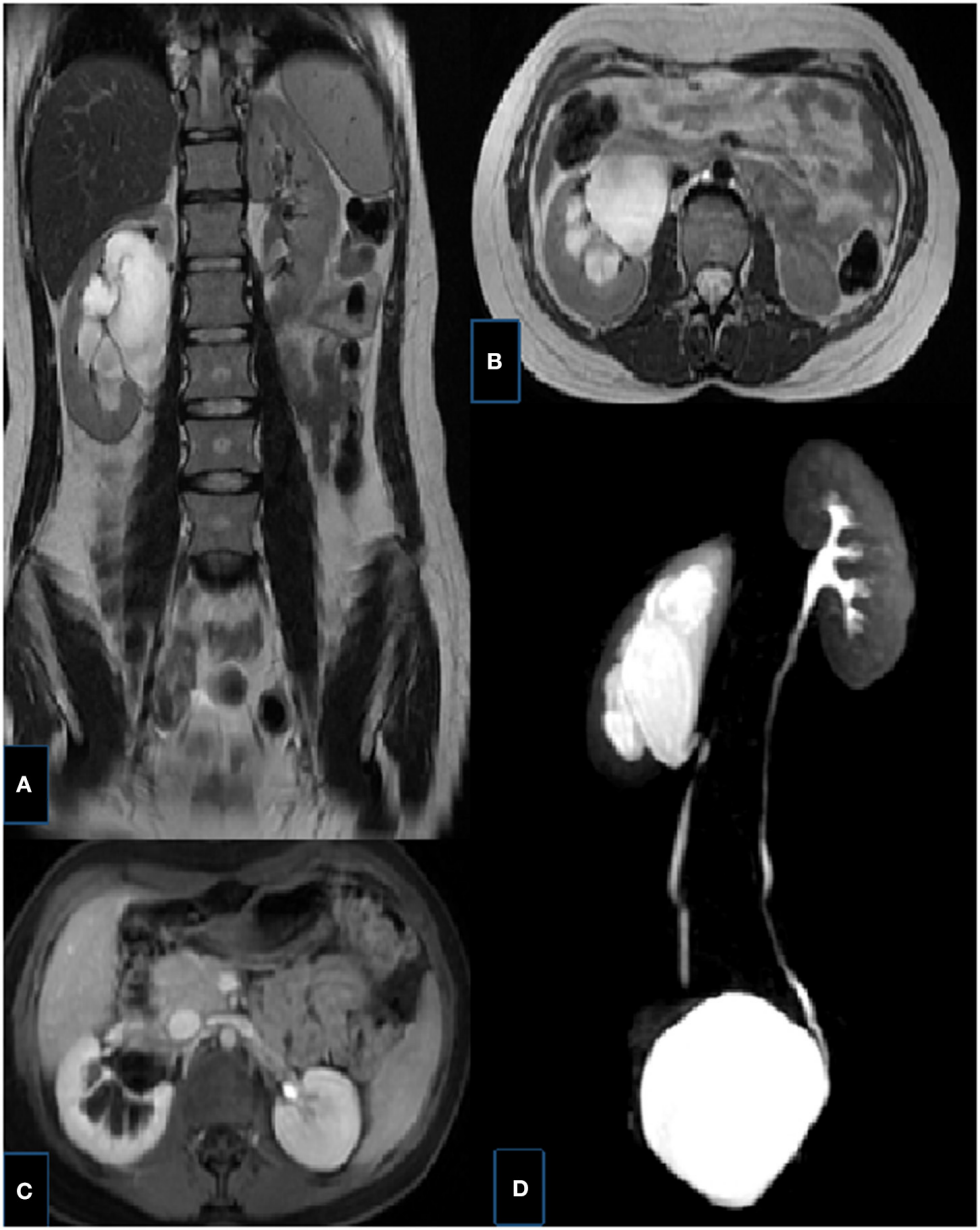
Right UPJ obstruction in a 14-year-old girl. (A,B) T2-weighted fast spin-echo coronal (a) and axial (b) MR images showing right renal collecting system dilatation, pelvis is extrarenally located, the thickness of renal parenchyma is decreased and corticomedullary differentiaton is lost. (C) Axial post-contrast excretory phase showing delayed excretion in the right renal collecting system, notice the contrast material in the left pelvis. (D) MIP MR image showing UPJ obstruction with kinking and angulation at the UPJ and a normal caliber ureter, left kidney is normal.
